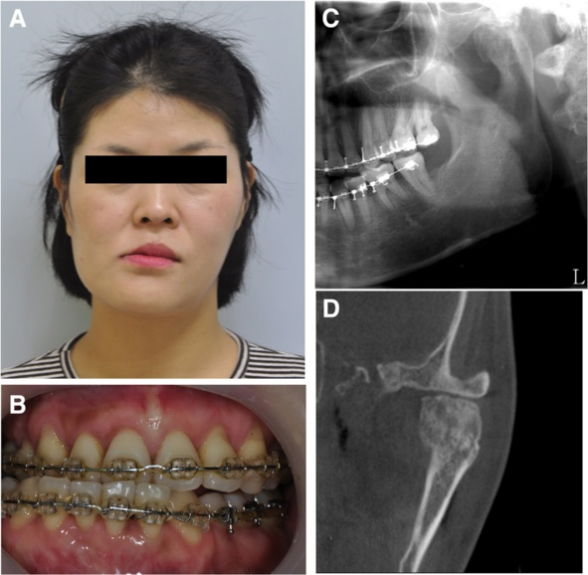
Pre-operative patient information. (A) The mandible was deviated to the right side in a clinical photograph. (B) The midline of the lower teeth was also deviated to the right side. (C) Panoramic radiograph shows the hypertrophic left mandibular condyle. (D) Cone-beam computed tomography image. A bony mass of irregular shape is detectable in the condyle.
